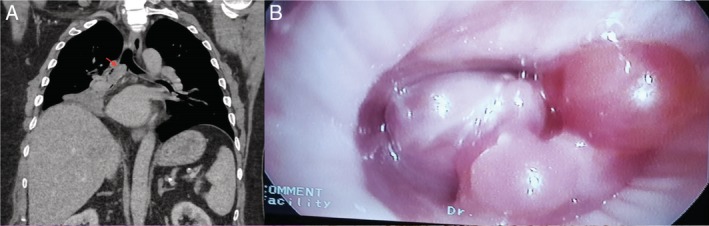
(A) Coronal reconstruction of computed tomography (CT) chest showing the bifurcation of trachea into right and left bronchial tree. Red arrow shows the endoluminal obstruction starting at the proximal right main bronchus and extending distally with lower lobe atelectasis. (B) Bronchoscopic image of multiple pink, smooth pedunculated lesions occluding the right main bronchus.
